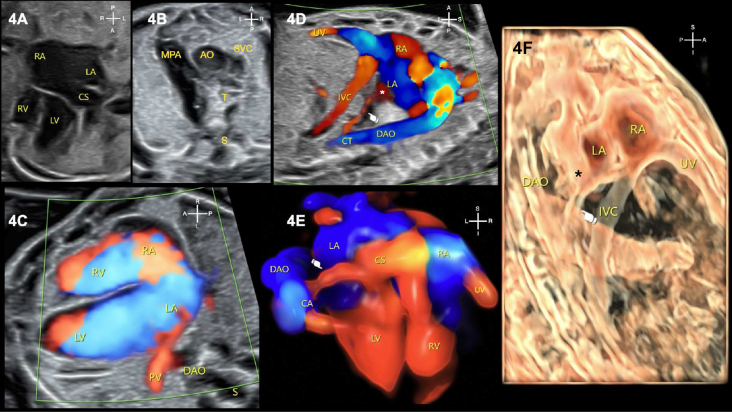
2-and 3-dimensional Fetal Echocardiography of Celiac Trunk Branch to Coronary Sinus Shunt With Umbilical Vein to Right Atrium Shunt in a 24 Weeks Fetus (A to F) CT branch–CS shunt with UV–RA shunt. (A) Four-chamber view showing dilated CS. (B) The 3-vessel view was normal. (C) Power Doppler 4-chamber view demonstrating normal pulmonary venous drainage. (D) Color Doppler sagittal view showing an anomalous branch from the CT draining into the CS. (E and F) Four-dimensional STIC power Doppler posterior 4-chamber view (E) and sagittal HD live tissue surface rendering showing the anomalous branch from the CT draining into the CS. ∗Coronary sinus. CT = celiac trunk; UV = umbilical vein; other abbreviations as in Figure 1, Figure 2, Figure 3.
